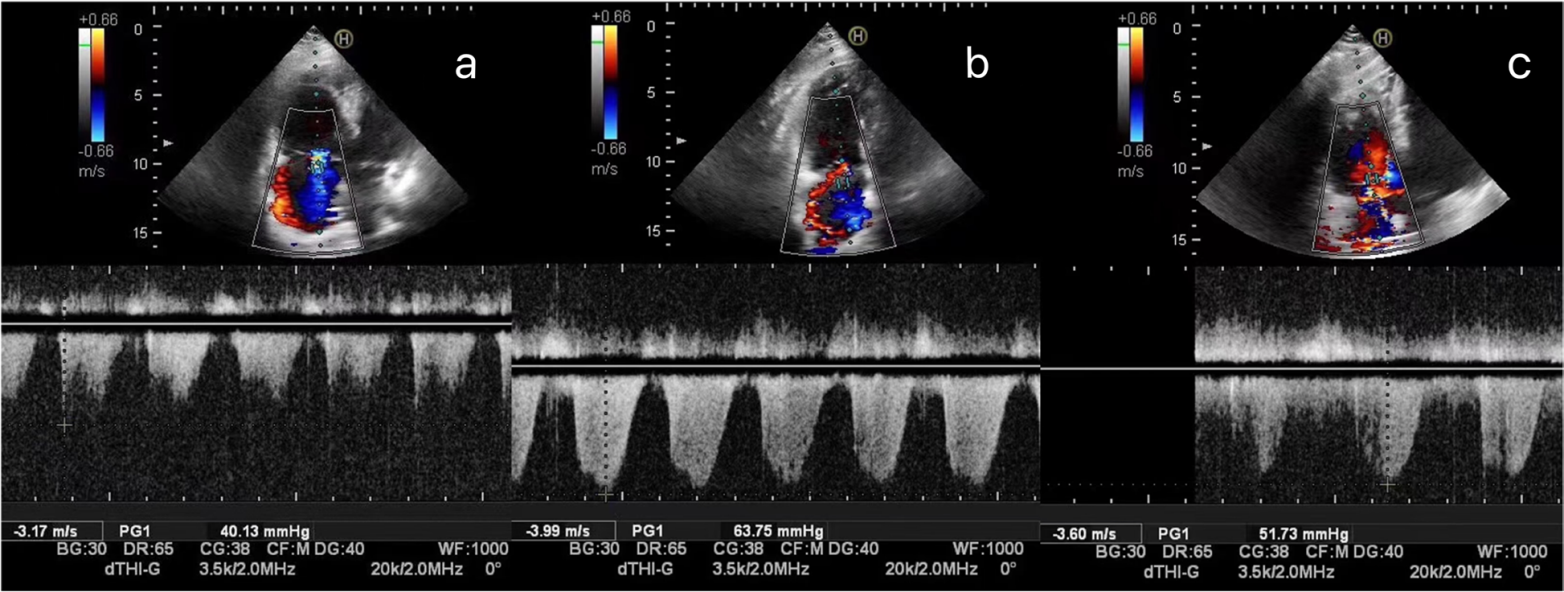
Parameters of a 60-year-old male patient with pulmonary hypertension, presenting with tricuspid regurgitation on color Doppler echocardiography. The systolic pulmonary artery pressure was estimated on days 3 (a), 8 (b), and 13 (c), using the gradient of tricuspid regurgitation pressure. The patient remained on extracorporeal membrane oxygenation support and exhibited low fever with stable heart rate and breathing
